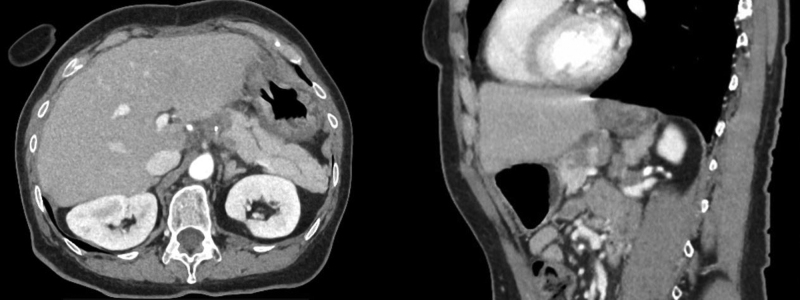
Abdominal CT showing a hypodense lesion of the pancreatic body. CT, computed tomography.
